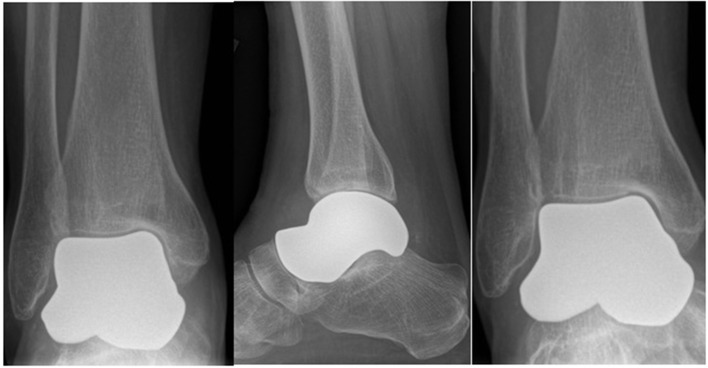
Post-operative 6 month radiographs (AP, lateral and mortise) of right total talus replacement.
